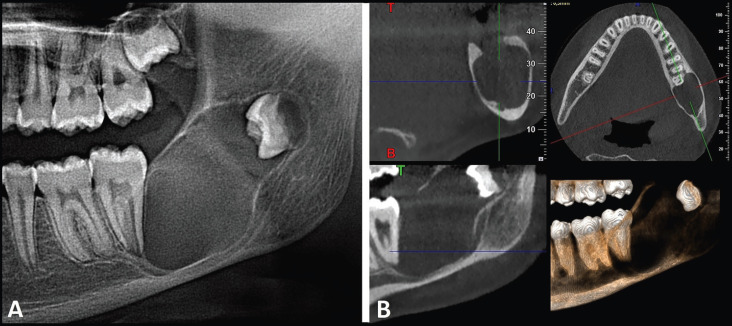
Radiological characteristics. A. Panoramic radiograph. B. Slides from cone-beam computed tomography.
